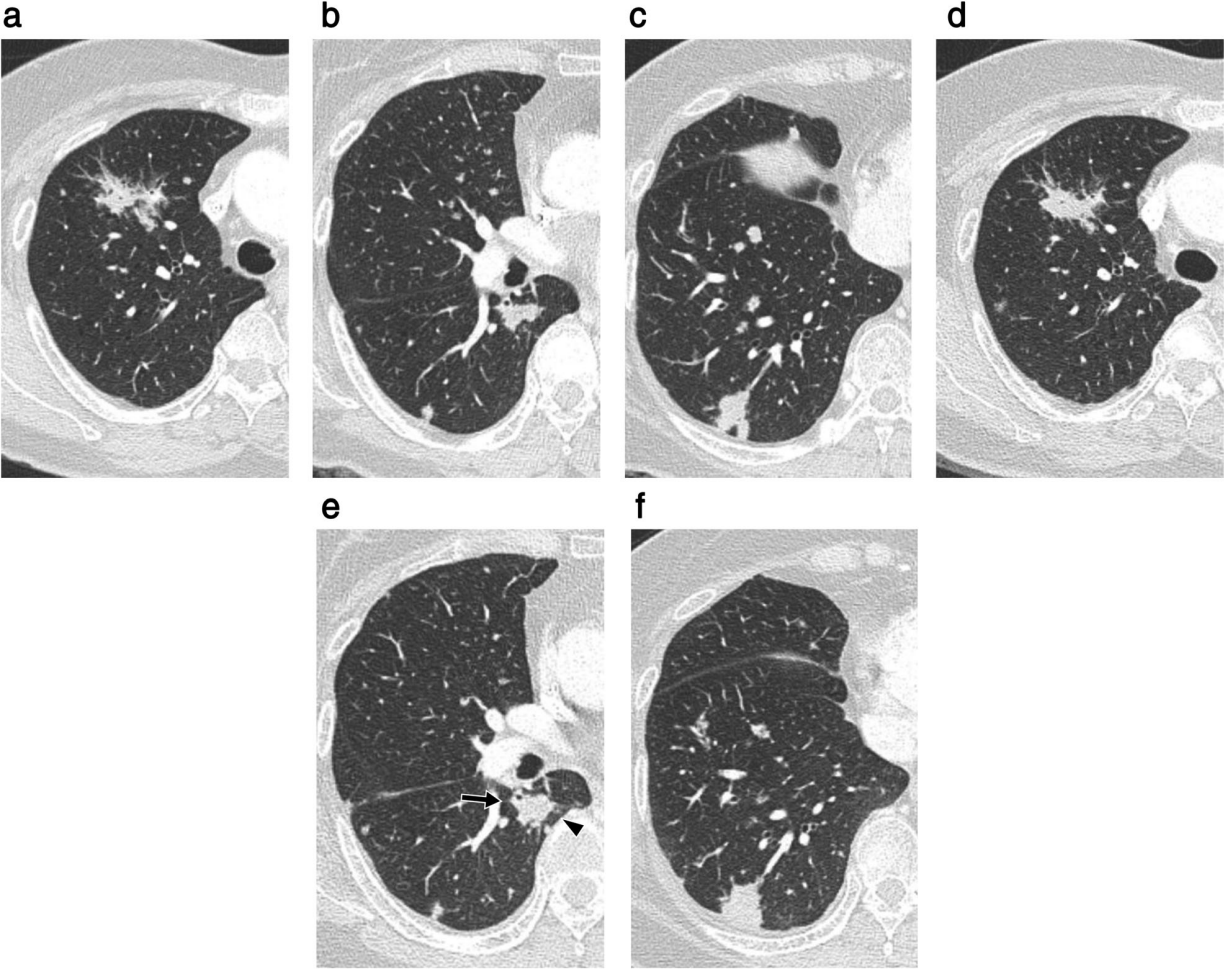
Axial chest CT images (section thickness, 3.0 mm) of a 64‐year‐old female patient with nonpneumonic‐type multifocal invasive mucinous adenocarcinomas in both lungs demonstrated spontaneous regression of airspace opacities (SRA). (a–c) Chest CT scan revealed (a) an irregular peribronchial lesion with surrounding ground‐glass opacity in the right upper lobe and (b, c) two irregularly‐shaped nodular cancer lesions in the right lower lobe. (d–f) After receiving two cycles of cisplatin‐based combination chemotherapy with doxorubicin, follow‐up chest CT showed (d) slightly decreased size of the peribronchial lesion in the right upper lobe with regression of the perilesional ground‐glass opacity, while showing (e) change in shape of another lesion in the right lower lobe – decreased in one portion (arrow) and increased in another portion (arrowhead). (f) Another irregularly‐shaped lesion in the right lower lobe had increased in size. This pattern of change after anticancer therapy was also considered as SRA, because increase or decrease in extent of airspace opacities appears to occur regardless of the use of anticancer therapy.
